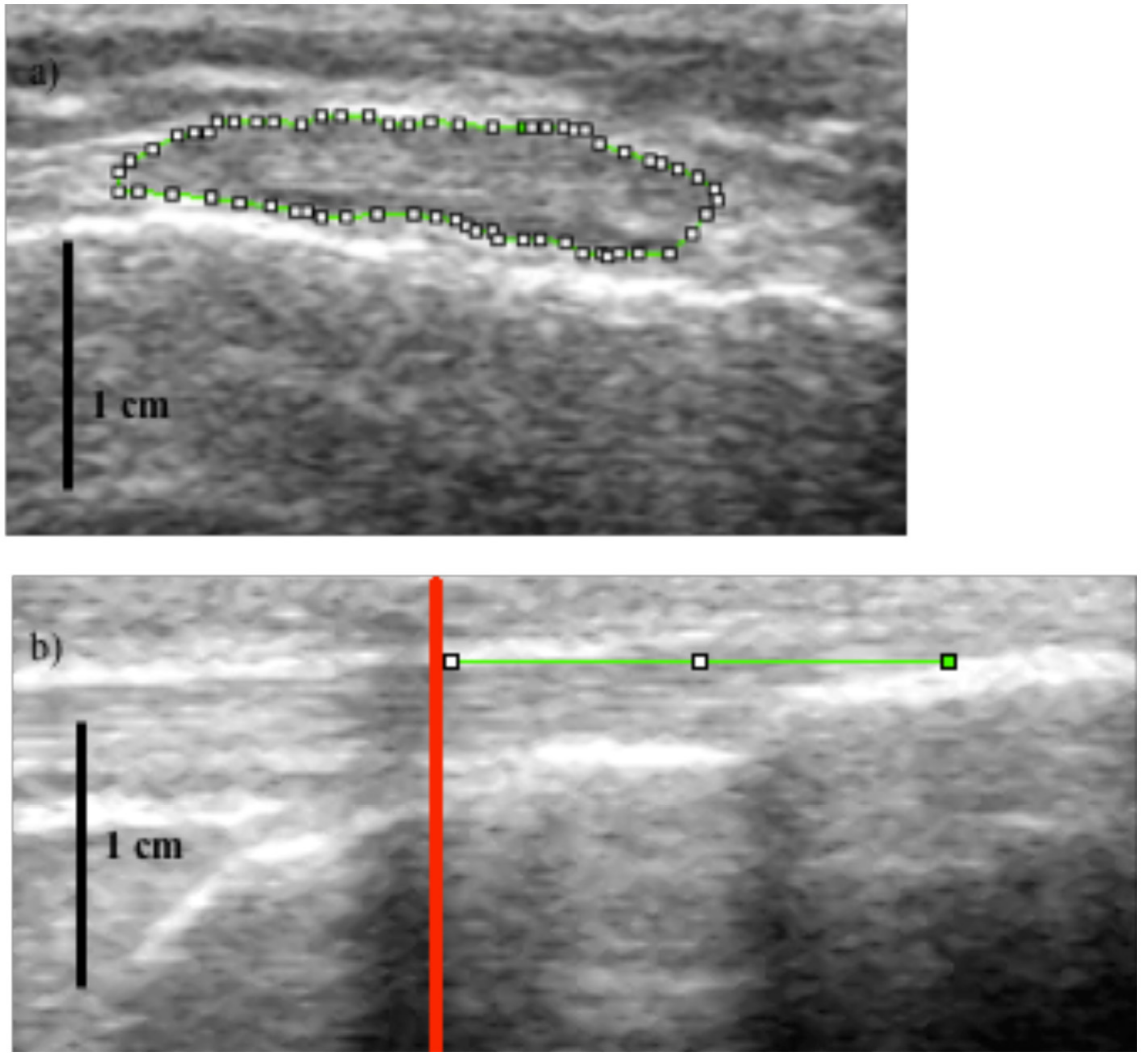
a) Example figure of measuring patella tendon cross-sectional area at 50% of patella tendon length using ultrasound. b) Example figure of measuring patella tendon lengthening using ultrasound. The red line emphasizes the shadow cast by the echo-absorptive marker on the skin, with the displacement of the proximal or distal anatomical marker made relative to this line. The distal insertion of the patella tendon into the tibia is shown in (b).
